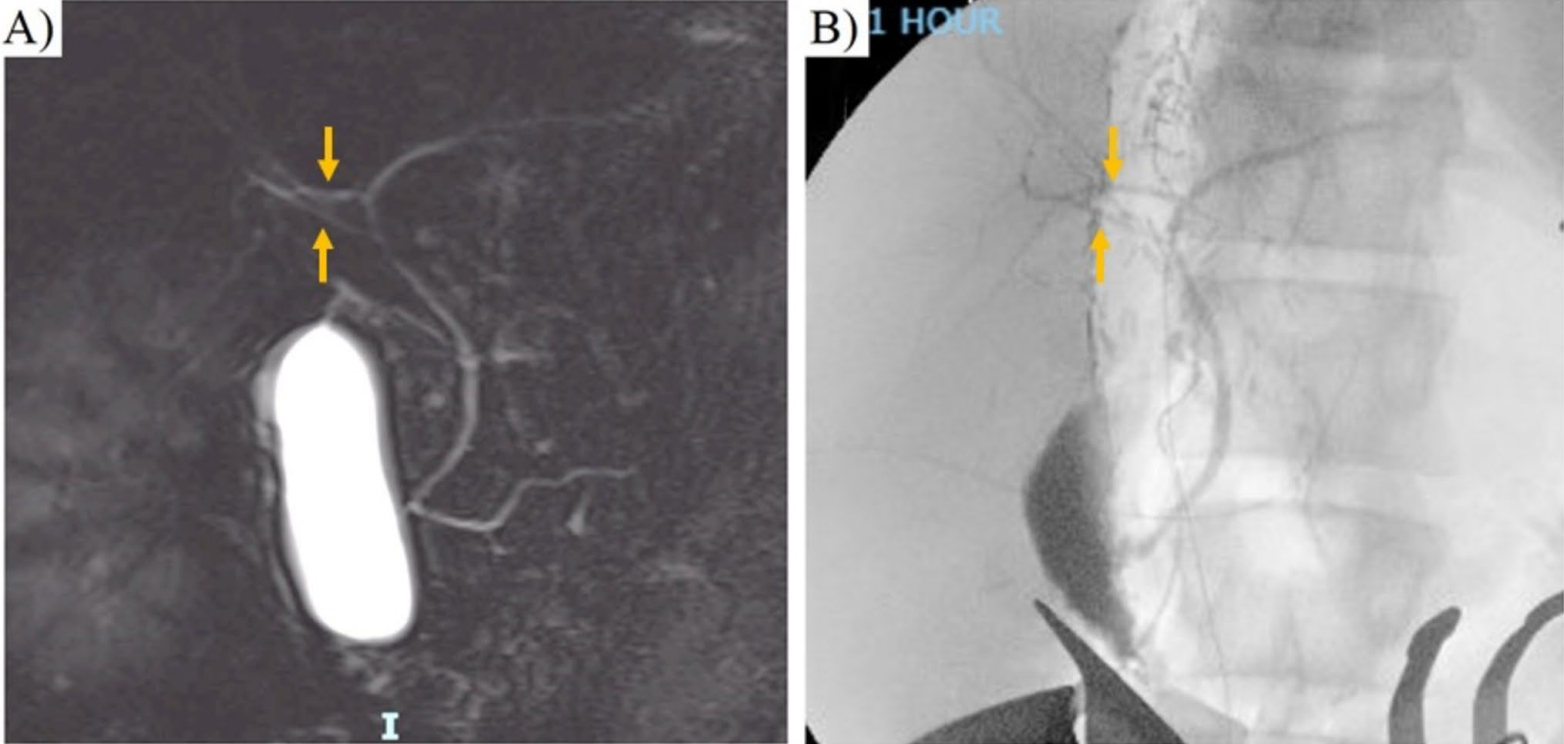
Pre-operative assessment with magnetic resonance cholangiopancreatography (MRCP) in a living donor candidate with two separate right hepatic ducts (A). Intraoperative cholangiogram of the same donor consistent with preoperative MRI findings with evidence of two right hepatic ducts draining separately into the main and left hepatic ducts (B). Arrows: right-anterior and right-posterior hepatic ducts
